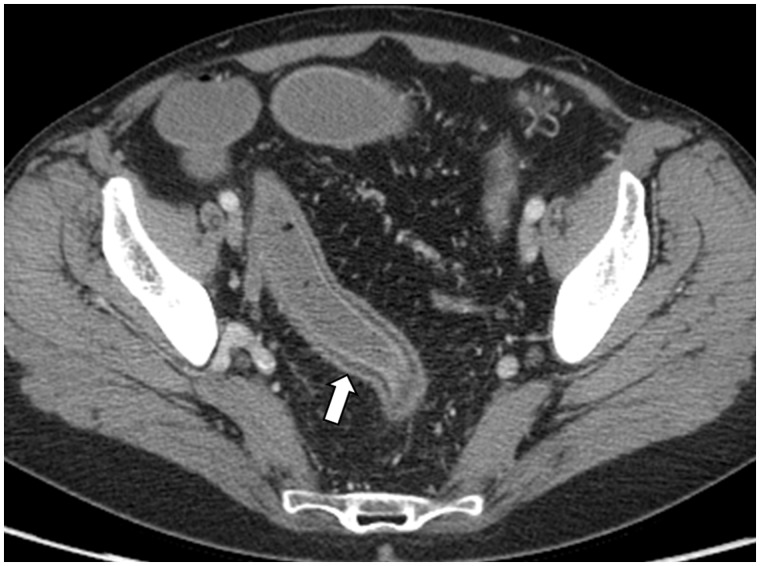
Computed tomography enterography in a 55-year-old female with pancolonic ulcerative colitis. White arrow highlights region with wall thickening and enhancement.
